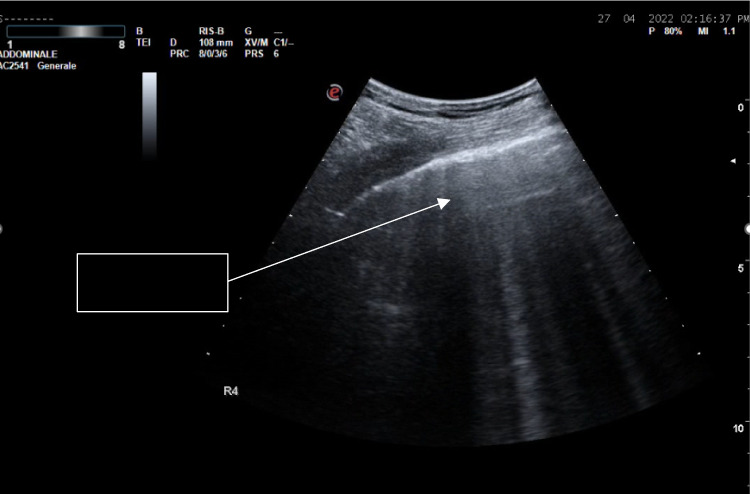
Lung Ultrasound (LUS) finding: B-lines in a patient with acute interstitial syndrome
